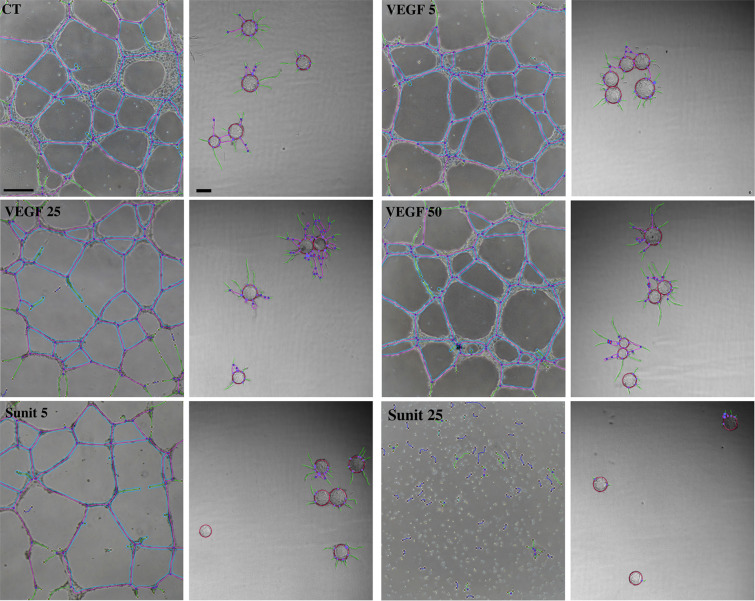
Comparative network patterns of HUVEC. ETFA (left column images) and FBA, (right column images). HUVEC were grown for 1 day (ETFA) or for 4 days (FBA) in the absence (CT, control) or presence of VEGF-A (5, 25 and 50 ng/ml) or of sunitinib (Sunit: 5 and 25 nM). Phase contrast images with the superposition of vectorial objects obtained from computer analysis using the customized “Angiogenesis Analyzer” for ImageJ are shown: green, *Branches*; magenta, *Segments*; red surrounded by blue, *Junctions*; cyan, *Meshes*; violet, *Anchorage Junctions*; red circle, *Sphere*. ETFA scale bar, left: 100 µm. FBA scale bar, right: 200 µm.
